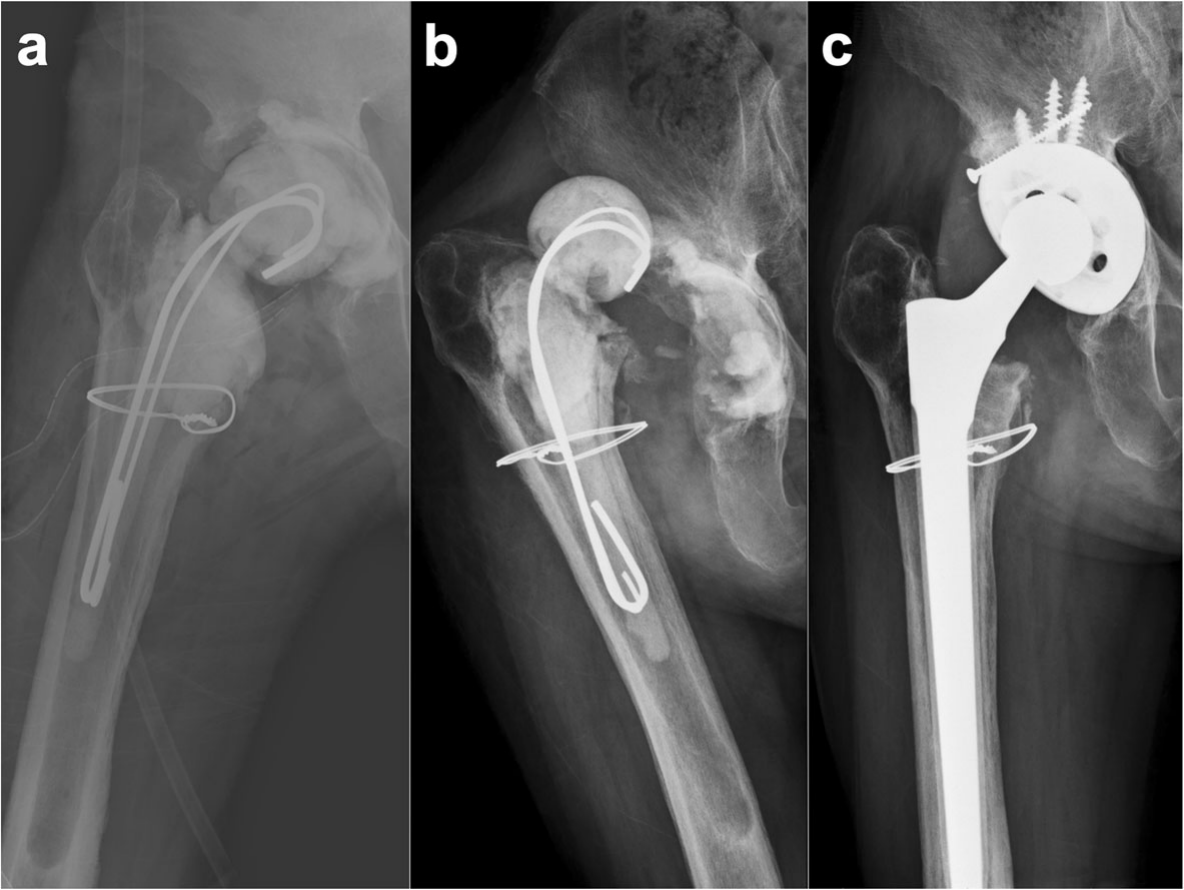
Hip radiographs showing: a) post-operative X-ray after implantation of a PMMA articulating spacer; b) dislocation of the PMMA articulating spacer 3 months later after implantation; and c) a well-fixed cementless total hip arthroplasty 2 years following reimplantation
